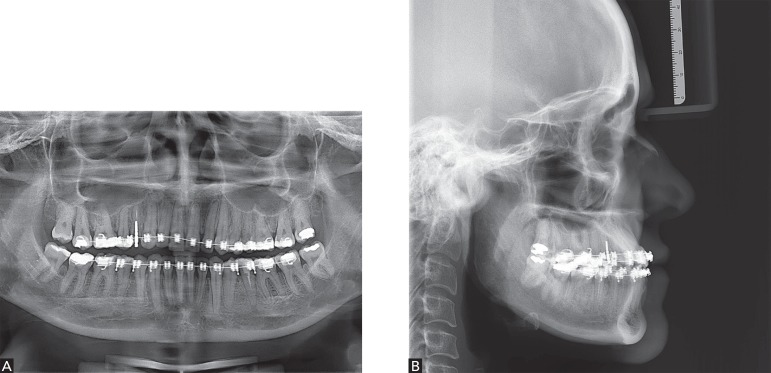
Radiographs at treatment completion: A) Panoramic; B)
